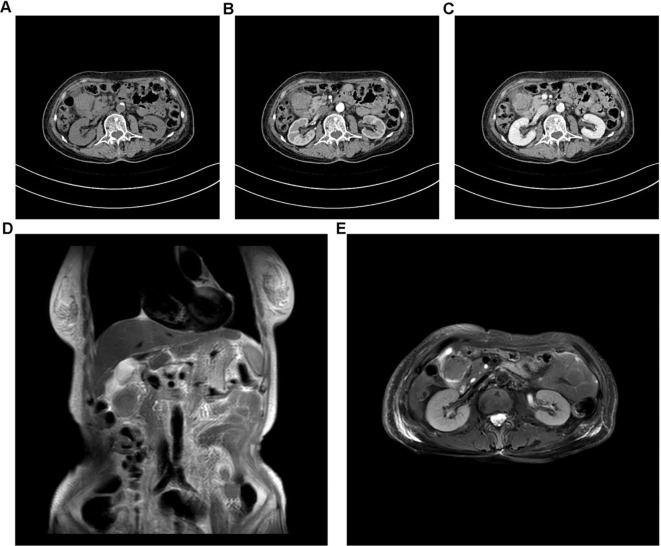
Imaging studies of the duodenal stump tumor. (A) Plain scan of the abdominal enhanced CT; (B) Arterial phase of the abdominal enhanced CT; (C) Venous phase of the abdominal enhanced CT; (D) Coronal section of the MRI; (E) Transverse section of the MRI.
